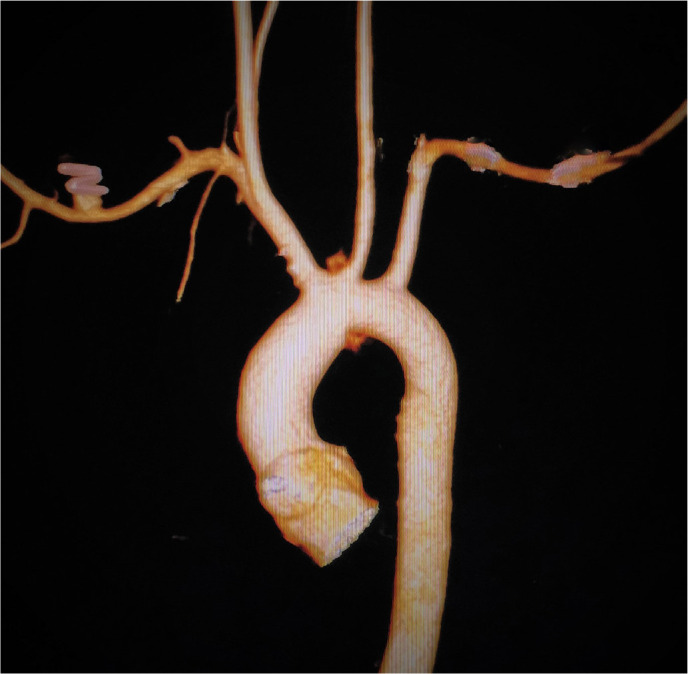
Postoperative CT scan showing almost normal arch with well-flowing
